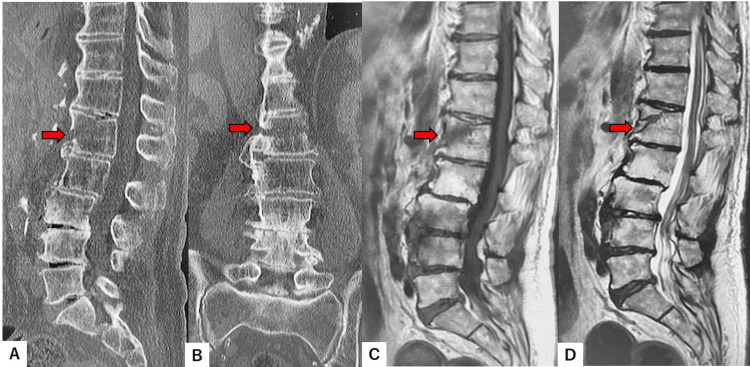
Preoperative images A: Mid-sagittal reconstruction CT. B: Coronal reconstruction CT. C: Mid-sagittal T1-weighted MRI. D: Mid-sagittal T2-weighted MRI. Red arrows indicate fracture line. CT: computed tomography, MRI: magnetic resonance imaging
